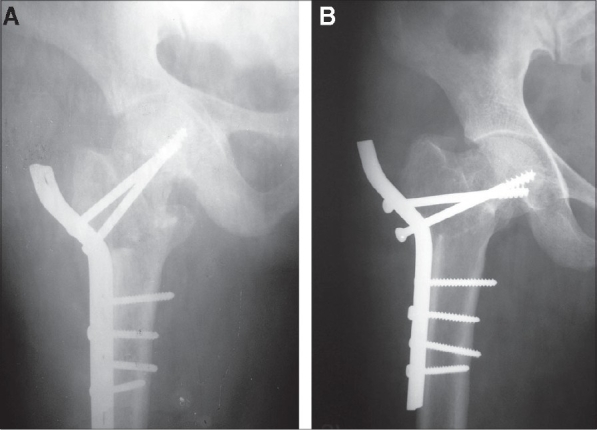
Coxa vara following inappropriate fixation. (a): X-ray antero posterior view showing the plate is not well contoured and standing away from the trochanter. (b): X-ray antero posterior view at four months. The fracture and osteotomy had united but the fracture drifted into coxa vara
